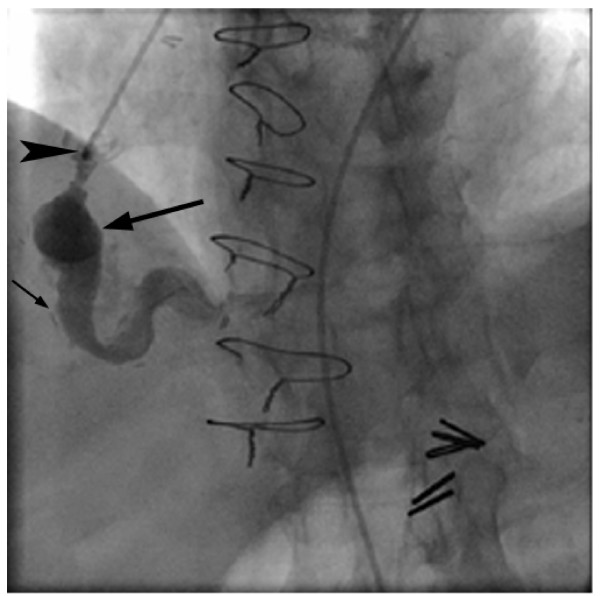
Selective injection of the aorta to RCA reverse saphenous vein graft. The tip of the catheter is seen at a mildly narrowed ostium (arrowhead), and the lumen of the saphenous vein graft aneurysm (large arrow) with areas of ulceration (small arrow) reproduce the findings seen previously at coronary CTA.
